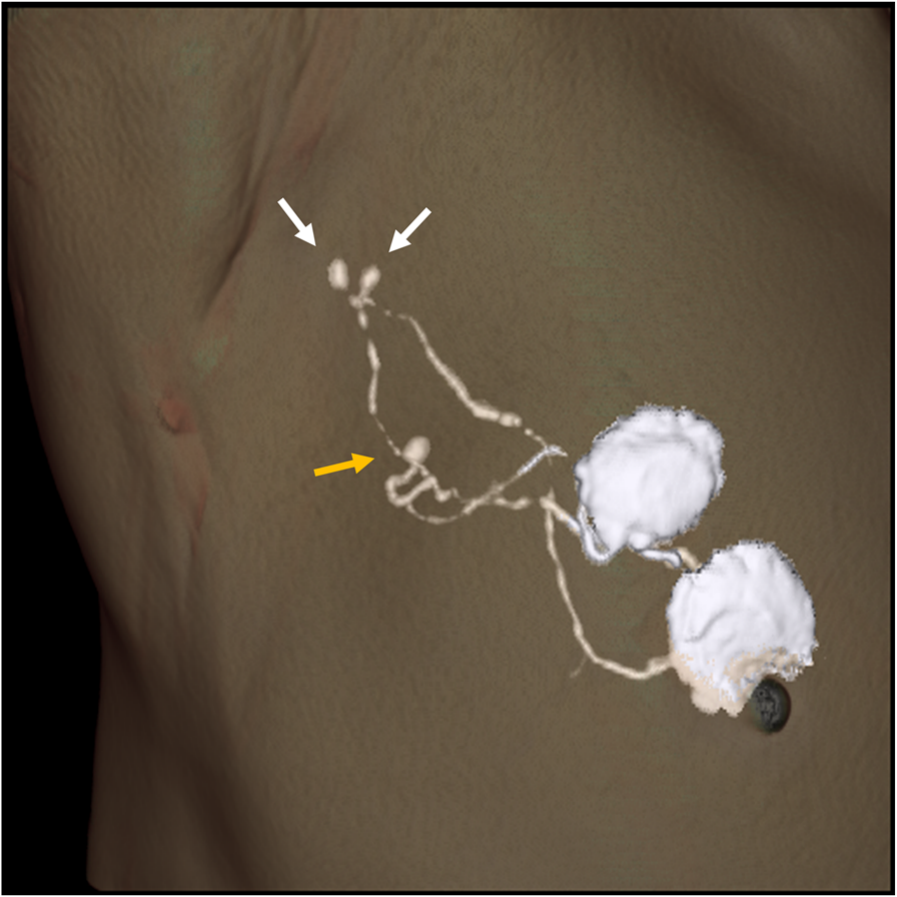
Three sentinel nodes were identified by computed tomography -lymphography (CT-LG). One (yellow arrow) might have been missed when sentinel node biopsy was performed without CT-LG because it was located far from the other two nodes (white arrows) and was influenced by radioactivity from the injection site.
